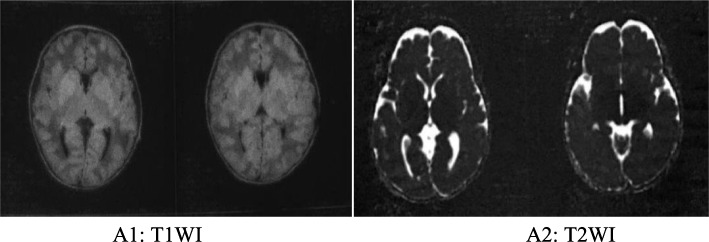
The MRI at the age of 14 days:DWI revealed that the cortical plate was slightly thin,the white matter area was relatively large, and the sulcus was slightly shallow. Symmetrical long T2 and DWI high-signal shadows were seen in the bilateral central gyrus, corona radiata, posterior limbs of internal capsules, thalamic region and cerebellar dentate nucleus
